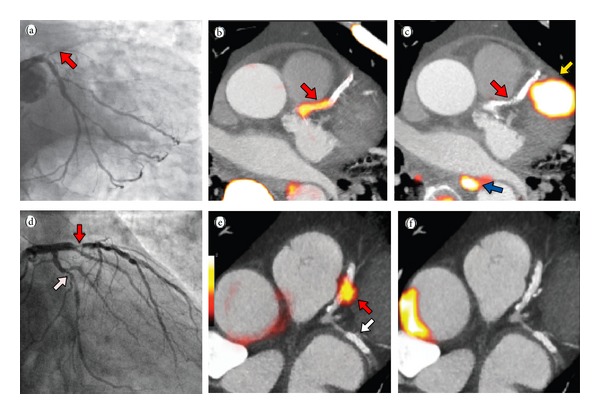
Acute STEMI patient with proximal LAD occlusion (red arrow) on angiography (a); subsequent ^18^F-NaF PET-CT imaging showing intense focal uptake in the culprit proximal LAD lesion (red arrow); (c) ^18^F-FDG PET-CT showing no uptake at the site of the culprit lesion (red arrow) with overlapping myocardial uptake of the culprit epicardial vessel; (d) Anterior NSTEMI patient with proximal LAD (red arrow) and LCx (white arrow) on angiography; focal intense uptake in only the culprit lesion (red arrow) on ^18^F-NaF PET-CT (e); and corollary ^18^F-FDG PET-CT showing no uptake in the culprit (LAD) or bystander (LCx) lesions (f) (reproduced with permission from Joshi et al. [86]).
